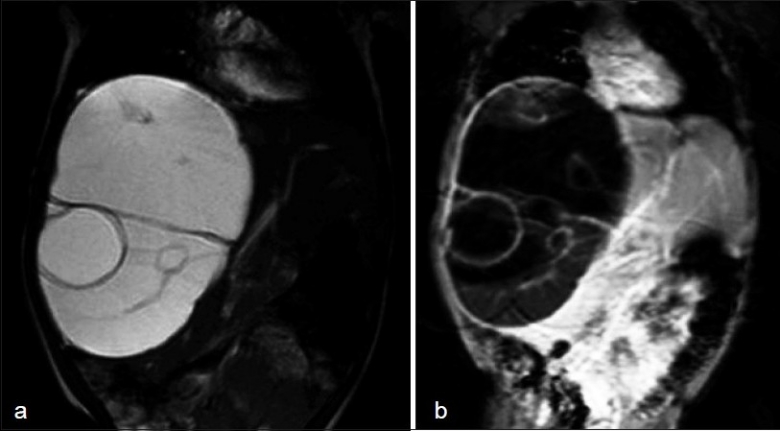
(a) MRI Abdomen (T2 weighted image), (b) MRI Abdomen (T1 weighted image)
